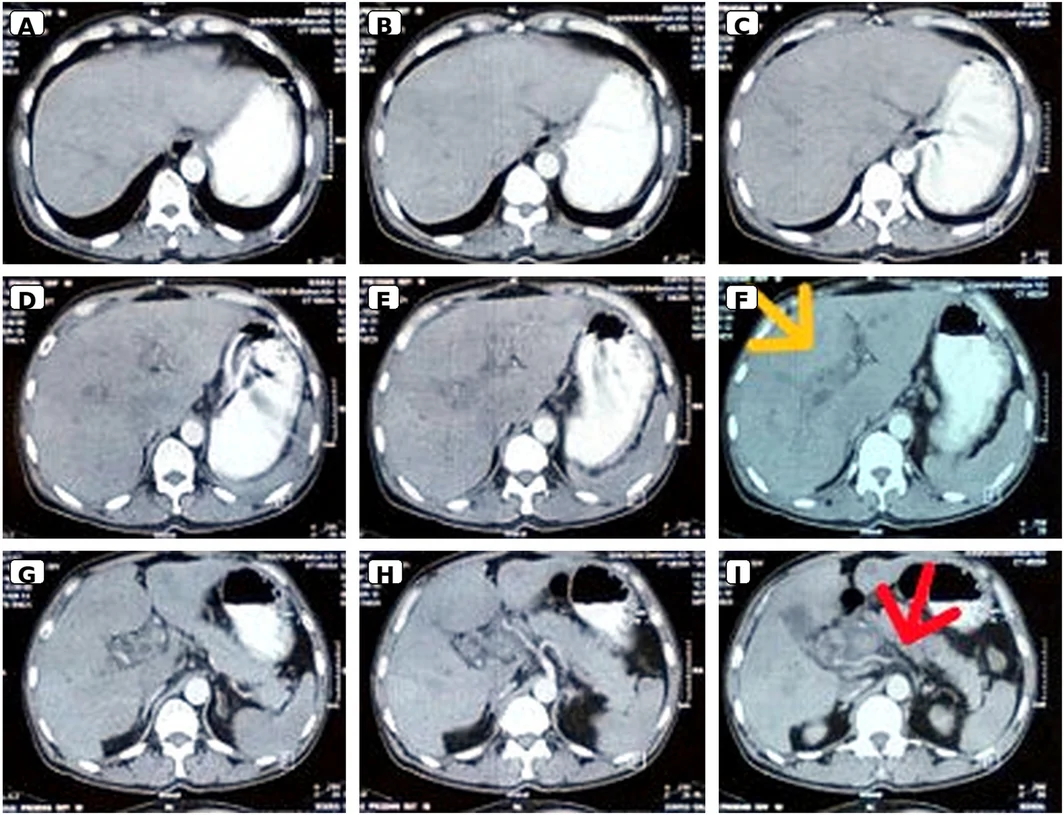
Contrast‐enhanced computed tomography (CT) of the abdomen. Panels A–I demonstrate portal vein thrombosis with periportal serpiginous collateral vessels, consistent with cavernous transformation. Red arrowhead: Thrombus in portal vein, Yellow arrowhead: Dilatation of CBD and intrahepatic biliary tree.
